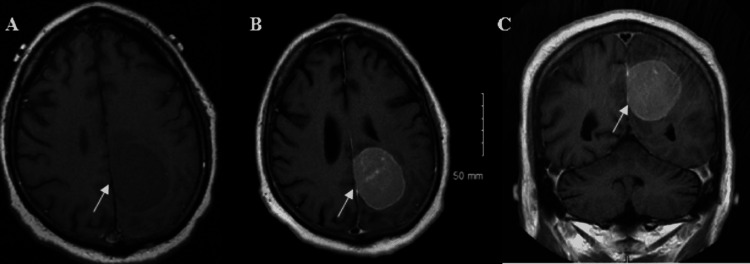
Initial MRI Brain with and without contrast. Noncontrasted T1 (A) and contrast-enhanced axial (B) and coronal (C) images show a homogeneously enhancing left frontoparietal mass.
